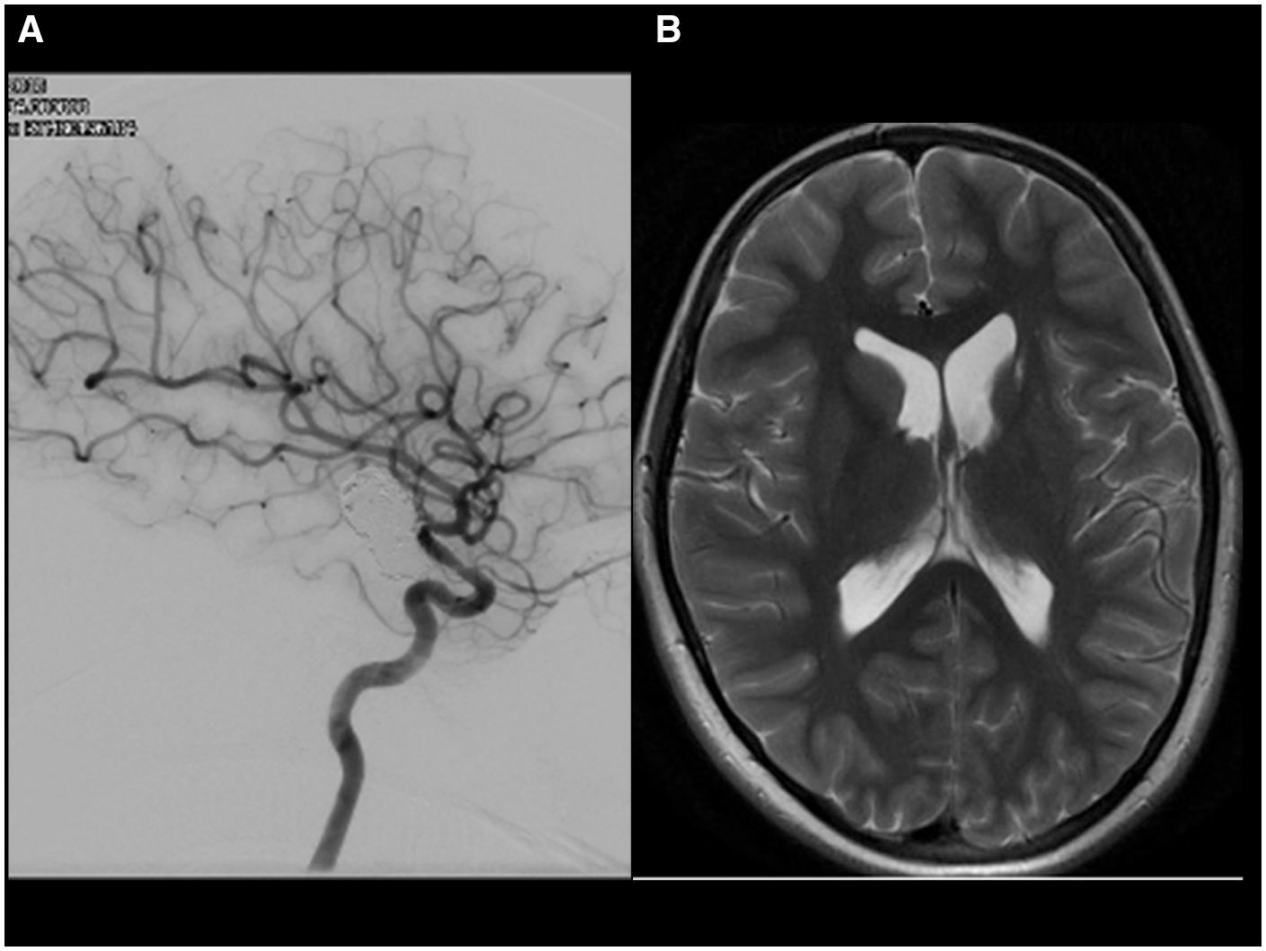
(A) The check angiogram 18 months after insertion of the flow-diverting stent shows aneurysm occlusion with a persistent in stent stenosis. (B) The MRI 12 months later and after discontinuation of all antiplatelet agents shows post ischaemic atrophy of the left-sided basal ganglia. The aneurysm has further shrunk. There was no new ischaemia (not shown).
